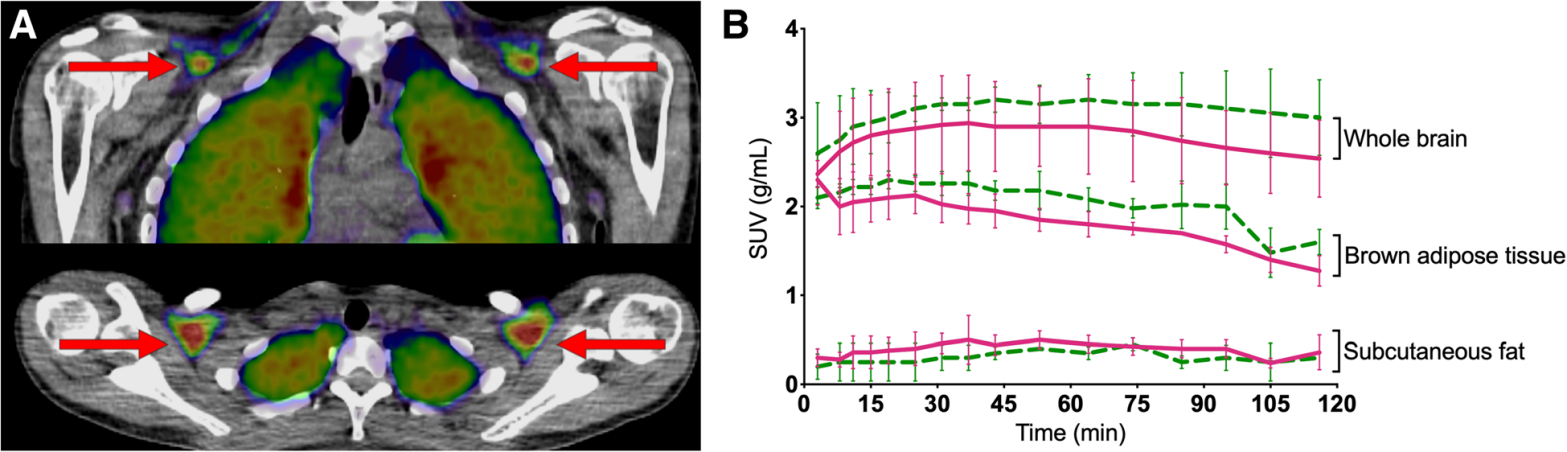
Anatomical localization of uptake in presumed brown adipose tissue (BAT) (a). Standard uptake value (SUV) curves for BAT, whole brain, and subcutaneous fat (b). Full line (pink) denotes ^11^C-Cimbi-36 and dotted line (green) denotes ^11^C-Cimbi-36_5
